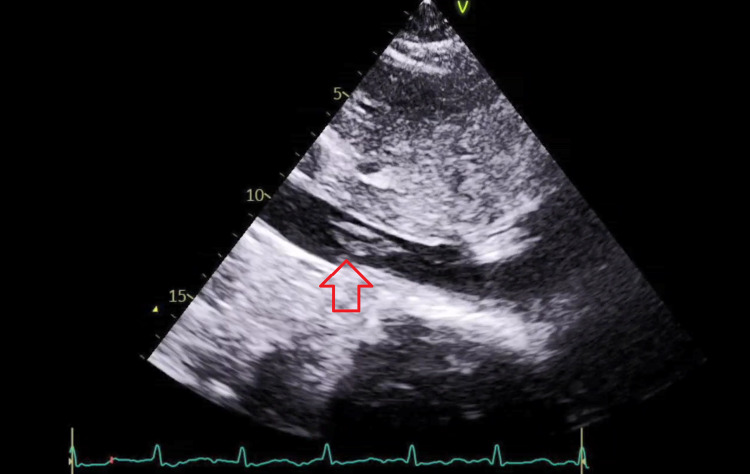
Bedside echocardiography showing IVC thrombus
